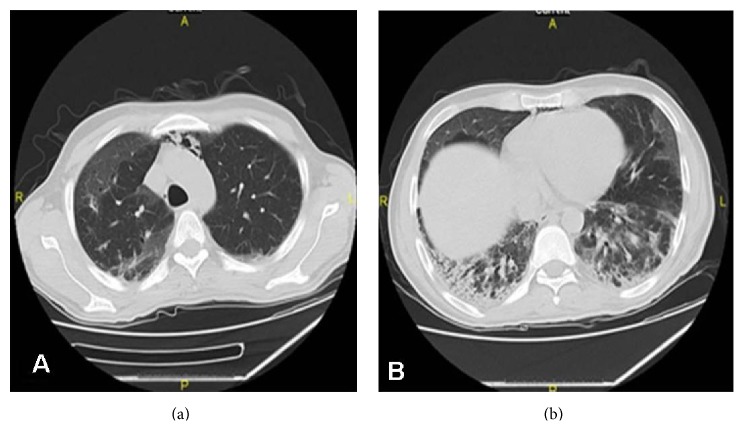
Thorax CT revealing bilateral ground glass opacity in subpleural areas, consolidation, and pneumomediastinum.
